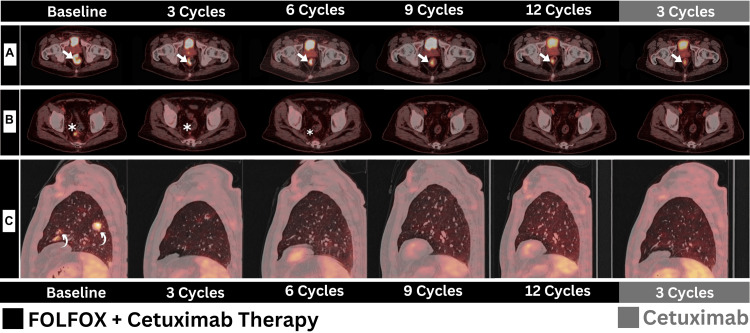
Serial fused PET/CT images captured from baseline and following each of the three cycles of therapy received A: A gradual regression in primary rectal malignancy was witnessed (arrows), reflecting both metabolic and morphologic responses on axial PET/CT images of the rectum. B: A similar response (asterisk) was also achieved for the few perirectal lymph nodes following the first six cycles, followed by a complete response. C: An earlier response was achieved for metastatic pulmonary nodules (visualized on sagittal PET/CT images), fading out after only three cycles of therapy (curved arrows) and maintaining complete resolution beyond that. FOLFOX - folinic acid, fluorouracil, oxaliplatin
